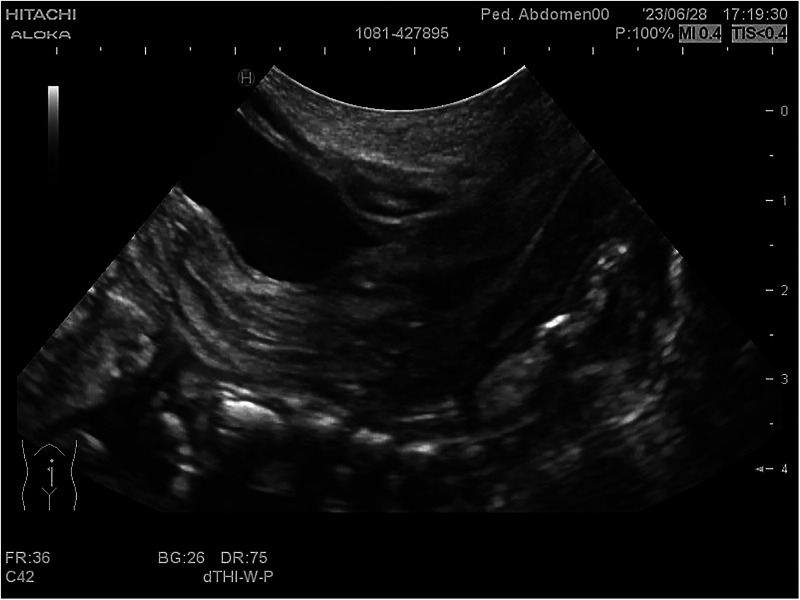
Pelvic and abdominal ultrassonography imaging of the second-born twin.
